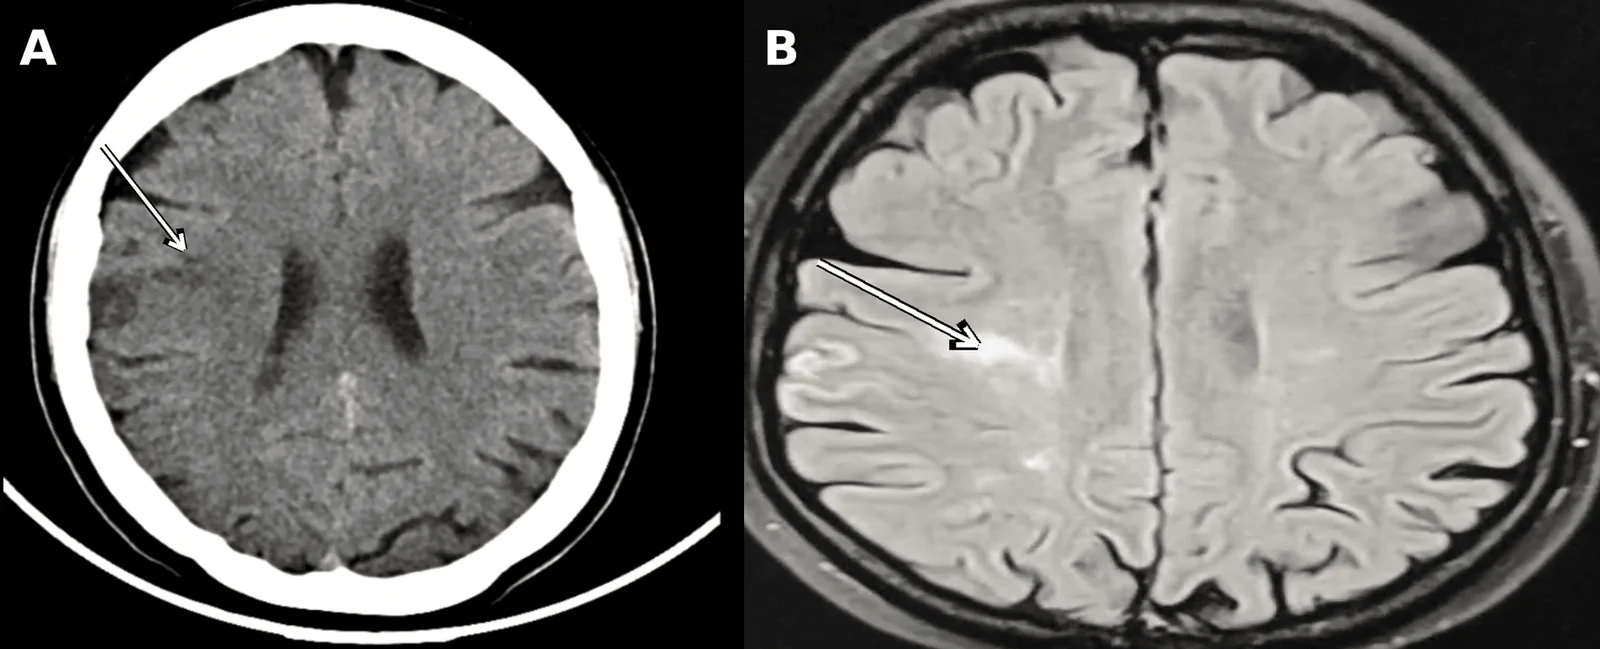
Neuroimaging findings at presentation (A) Non-contrast axial head CT obtained on day 1 after symptom onset, showing a subtle hypodense area in the right frontal white matter (arrow). (B) Contrast-enhanced axial fluid-attenuated inversion recovery MRI obtained on day 6, showing a corresponding area of increased signal in the posterior right frontal region with cortical involvement and extension into the adjacent deep white matter (arrow). The formal radiological report additionally documented gyriform enhancement and restricted diffusion, consistent with a subacute ischemic infarct.
